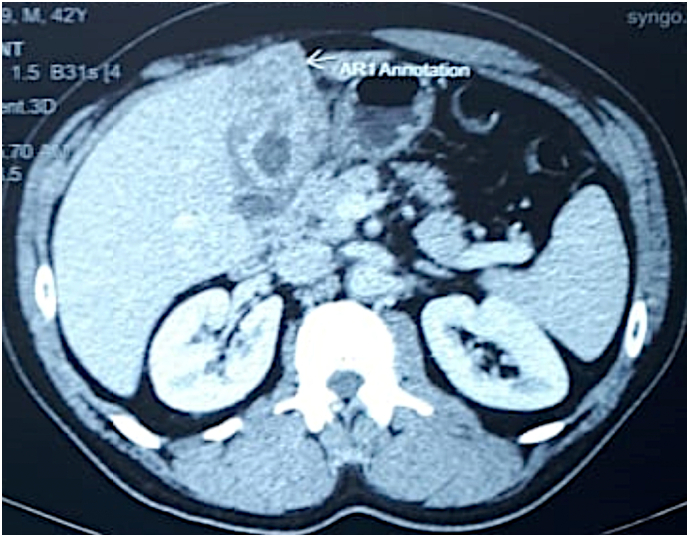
CT axial slice showing diffuse parietal thickening of the gallbladder with invasion of segment V and antropyloric region of the stomach.
